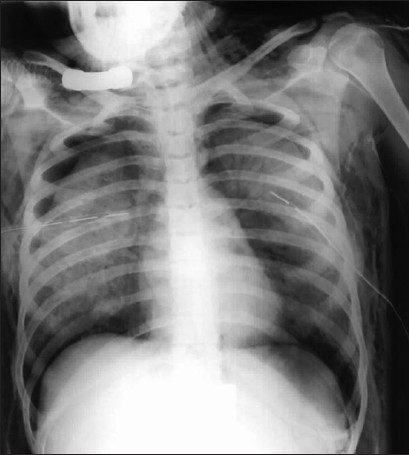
AP radiograph of the same patient with ARDS as in Figure 6 with further complication of bilateral pneumothoraces secondary to pleural drain placement
